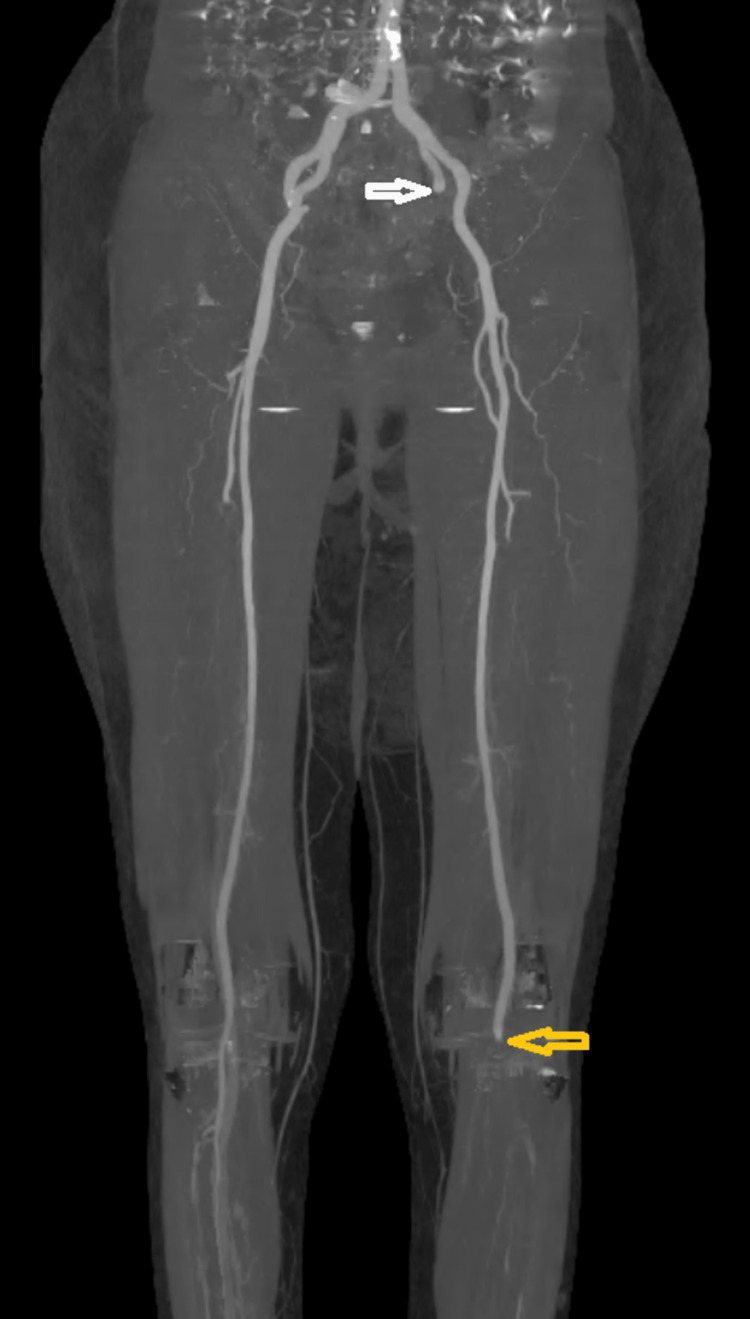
3D CT angiography revealing a left hypogastric (white arrow) and popliteal (yellow arrow) artery occlusion
